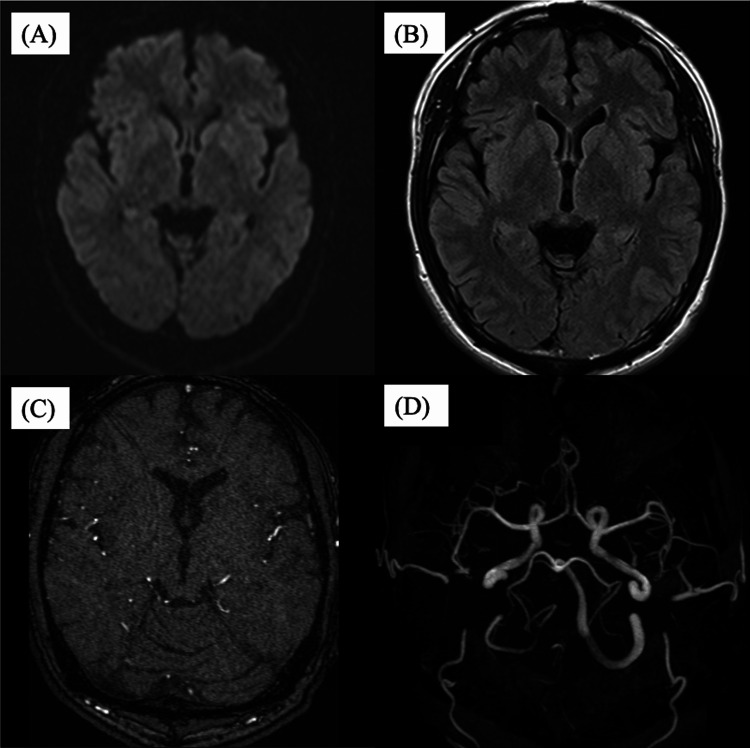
Follow-up MRI and magnetic resonance angiography (MRA) performed six months after treatment. (A) MRI diffusion-weighted imaging (DWI) showed no abnormal findings. (B) MRI fluid-attenuated inversion recovery (FLAIR) showed no abnormal findings. (C) TOF-MRA axial scan demonstrated absence of abnormal flow signal in the left lateral sinus. (D) TOF-MRA 3D reconstruction demonstrated absence of abnormal flow signal in the left lateral sinus.
